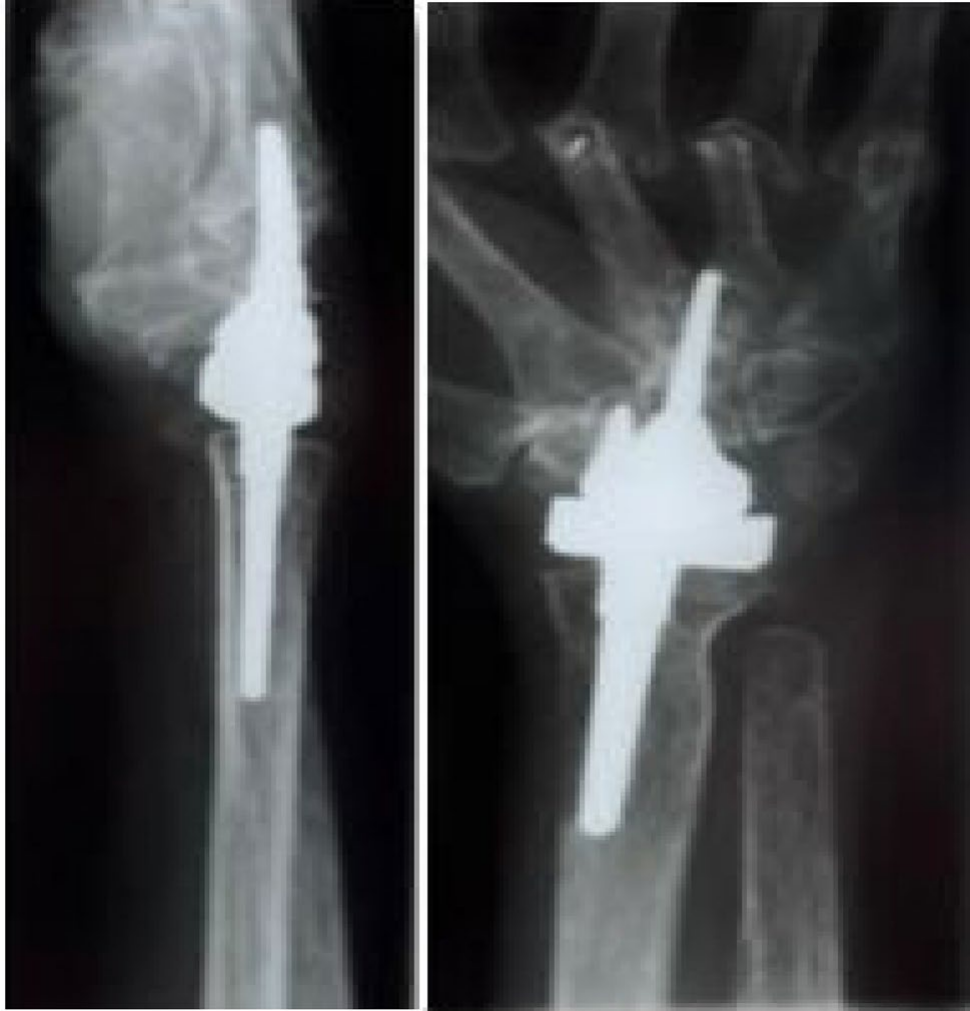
Case 4– Right postoperative X-ray in lateral and a.p. view
